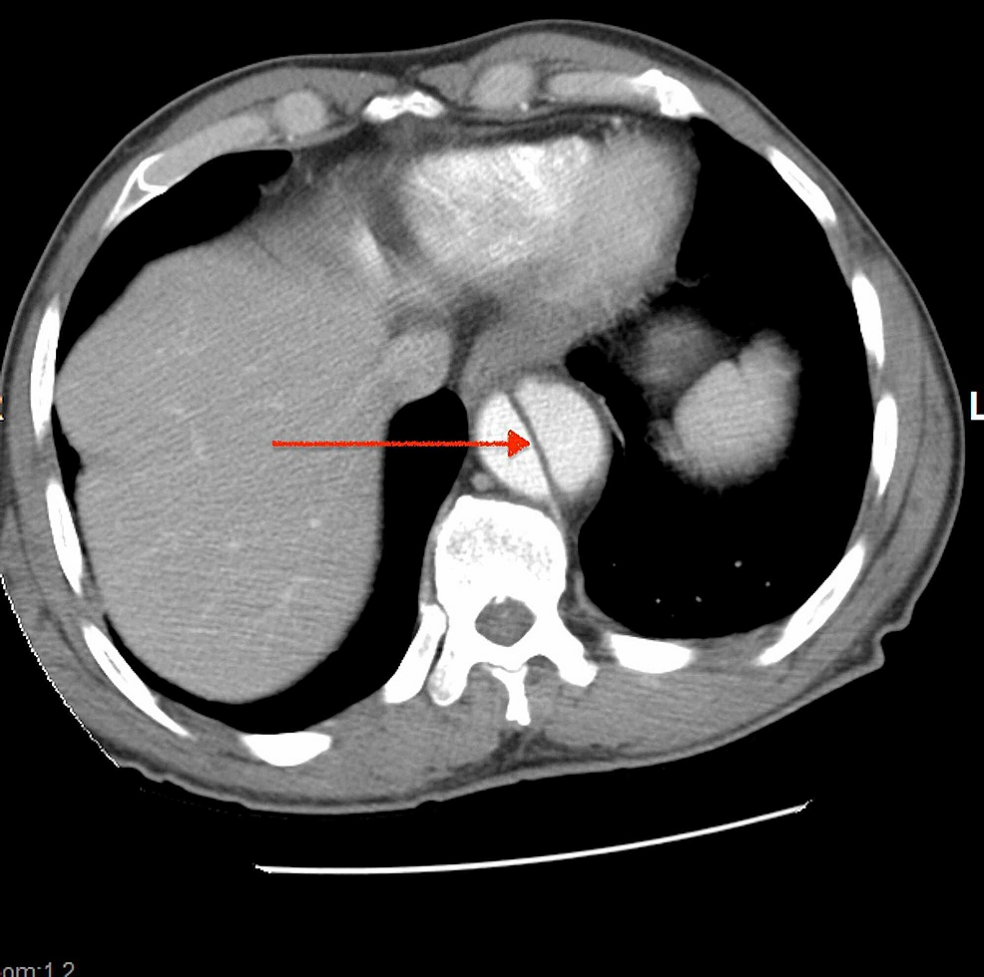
CT abdomen showing type B aortic dissection (supra renal) with true and false lumens separated by an intimal flap.
